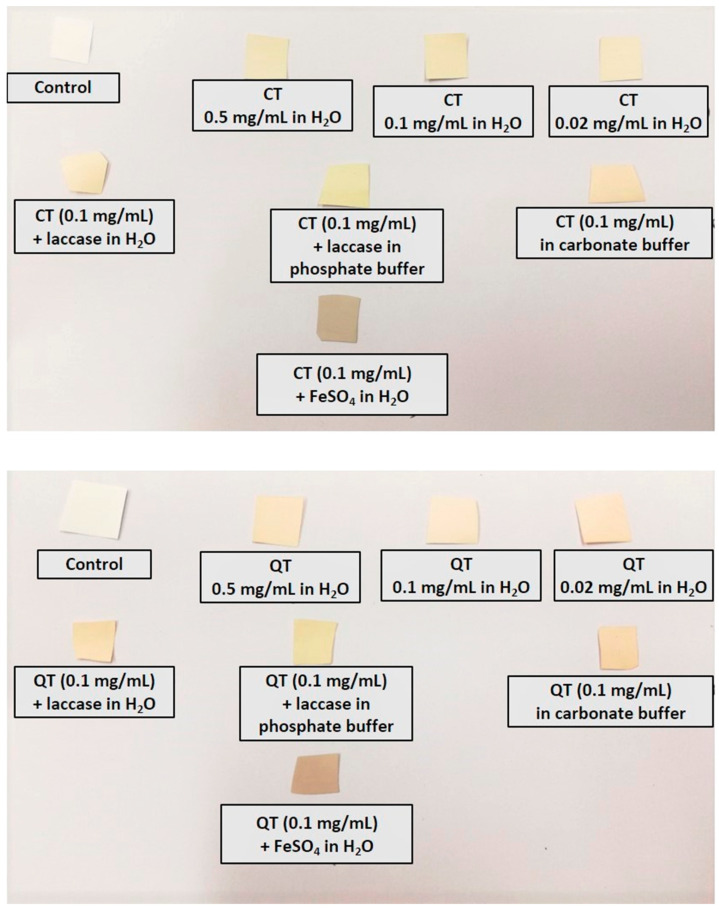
Nylon membrane filters coated with chestnut tannins (CT) (top) and quebracho tannins (QT) (bottom) under the different dip coating conditions explored.
